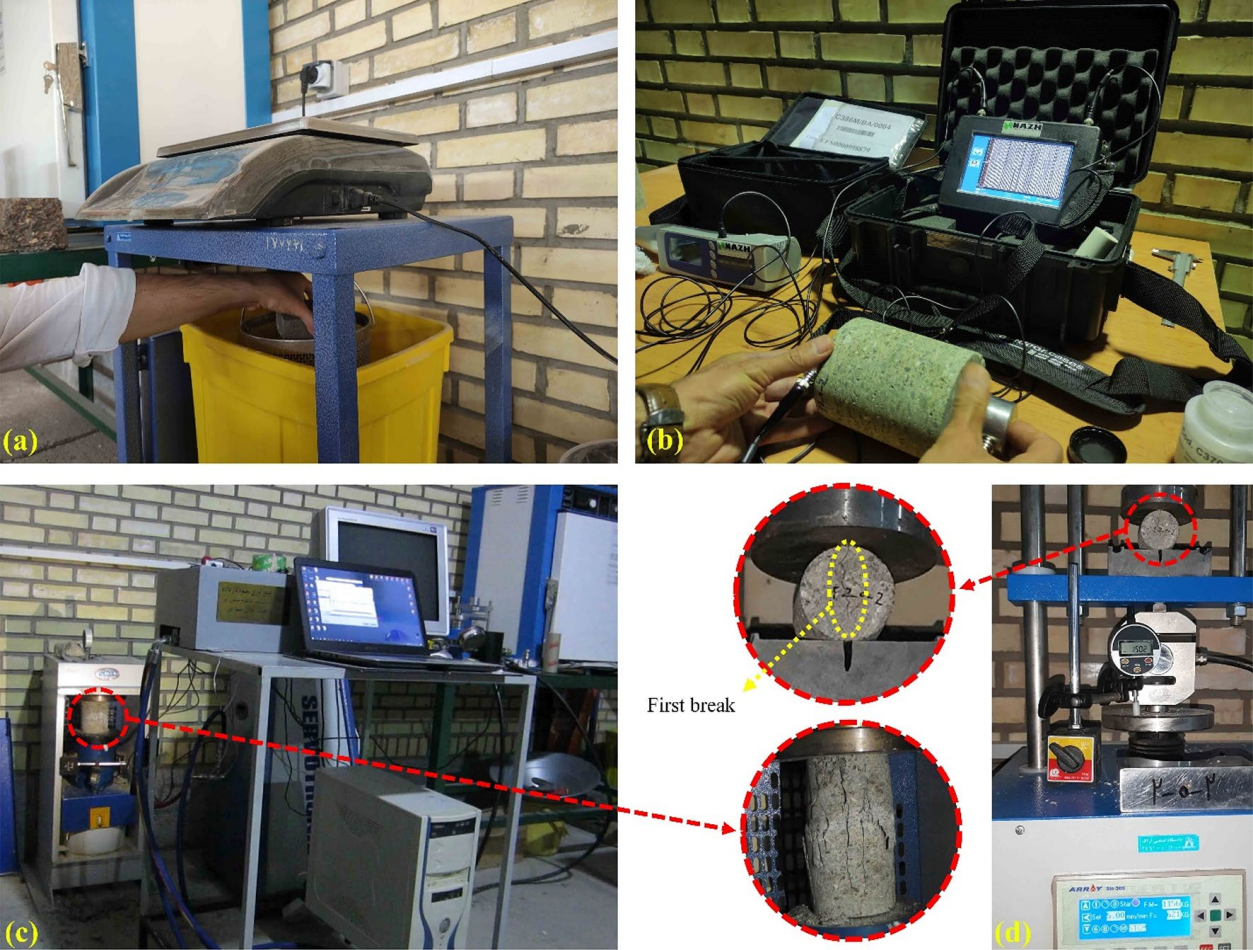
A view of the setup and testing methods, (a) physical propertie tests, (b) ultrasonic pulse velocity tests, (c) uniaxial compressive strength tests, (d) splitting tensile strength tests.
